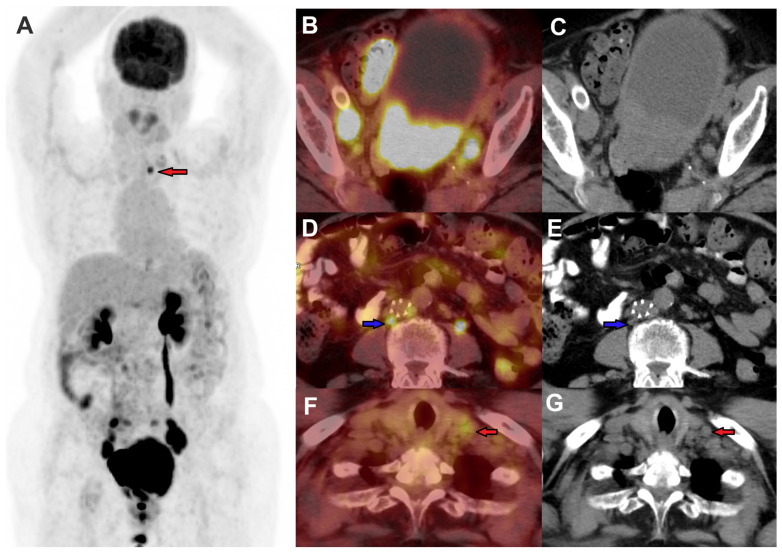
FDG PET/CT was performed in a female for a stage IVB cervical cancer staging, MIP image (A), axial fused PET/CT, and CT images demonstrating FDG-avid primary cervical lesions, with FDG-avid metastases to bilateral pelvic nodes (B,C), retroperitoneal nodes (blue arrow) (D,E), and a small left supraclavicular node (red arrows) (F,G).
